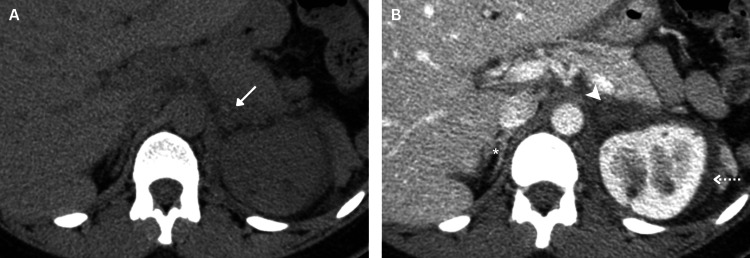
Follow-up contrast-enhanced CT performed five days later. (A) Unenhanced CT shows marked enlargement of the left adrenal gland (maximum limb thickness: 13 mm vs. 3 mm contralaterally) (arrow). (B) Portal venous phase demonstrates a complete absence of enhancement of the left adrenal gland (arrowhead) with surrounding periadrenal fat stranding (dotted arrow). The gland shows heterogeneous attenuation with low-density areas (~17 HU) and residual faint enhancing components (~75 HU) (not shown in this slice), compared with the contralateral adrenal gland (~100 HU) (asterisk).
